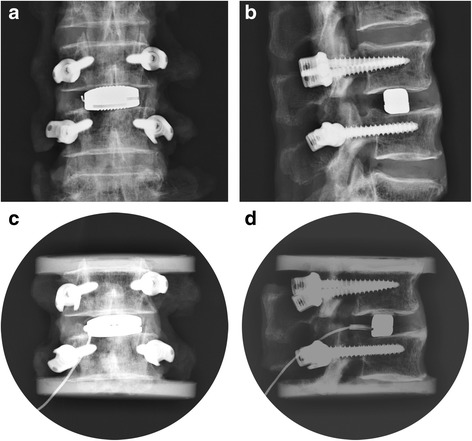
Cage position examined by X-ray. A long cage for flexibility test: AP view (a) and lateral view (b); A long cage for load sharing test, AP view (c) and lateral view (d)
